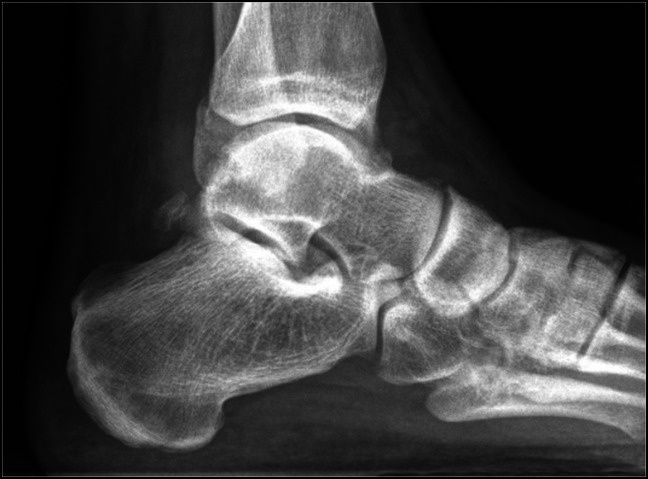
Lateral weight-bearing radiograph image 6 months postreduction showing maintained joint alignment and posterior fibula fracture union.
